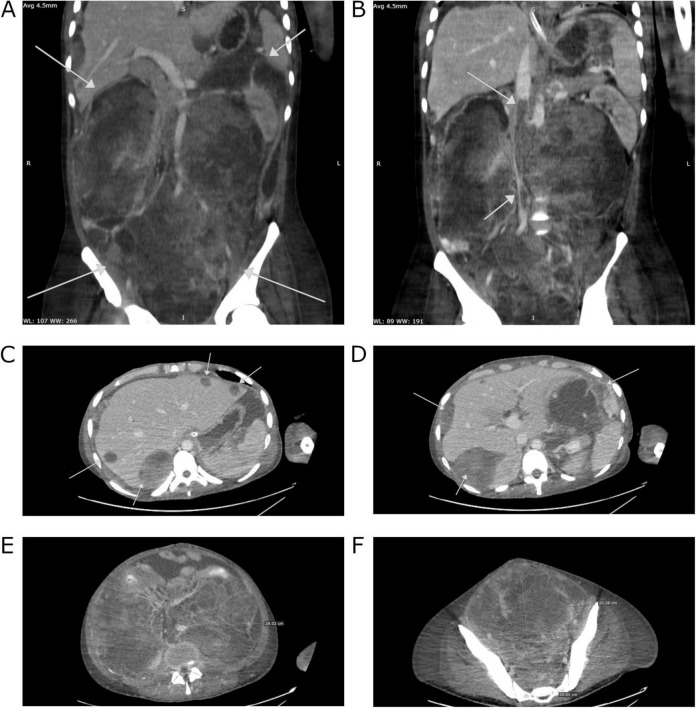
Computed tomography images of the patient’s abdomen from 31.12.2019 performed at The Maria Sklodowska-Curie National Research Institute of Oncology in Warsaw. (A) A mass occupying the entire abdominal and pelvic cavity. (B) Liver metastases. (C) Peritoneal metastases. (D) Thrombosis in the inferior vena cava (IVC). (E) Tumor mass in cross-section at the level of the mid-abdomen. (F) Cross-section at the level of the pelvis.
